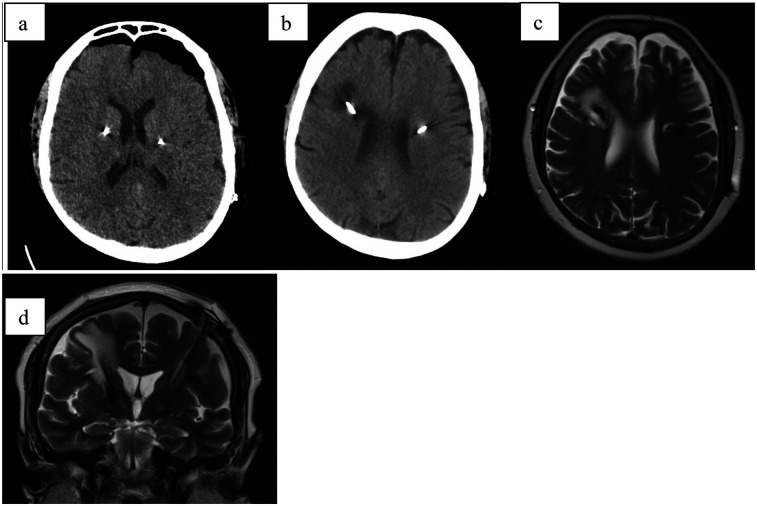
(a) CT scan directly after electrode implantation; (b) CT scan 4 weeks after implant; (c) MRI-T2 axial scan 4 weeks after stimulation initialization showing hyperintensive tissue surrounding the right electrode (d) MRI-T2 coronal scan 4 weeks after implant.
